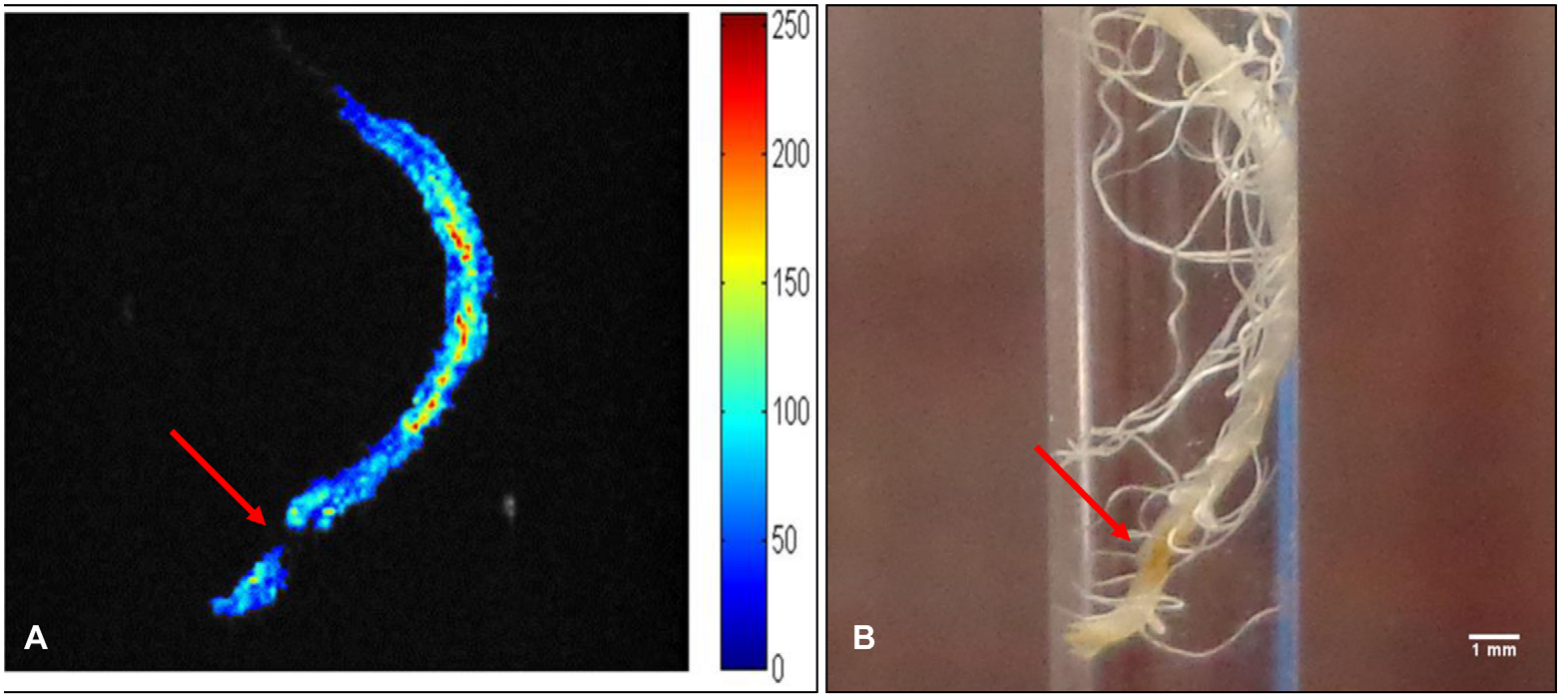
MRI image of rice root immersed in 5-DTPA for 6 h (A). Aging or dead tissues is marked with arrows. Image B shows a photograph image of the root sample.
